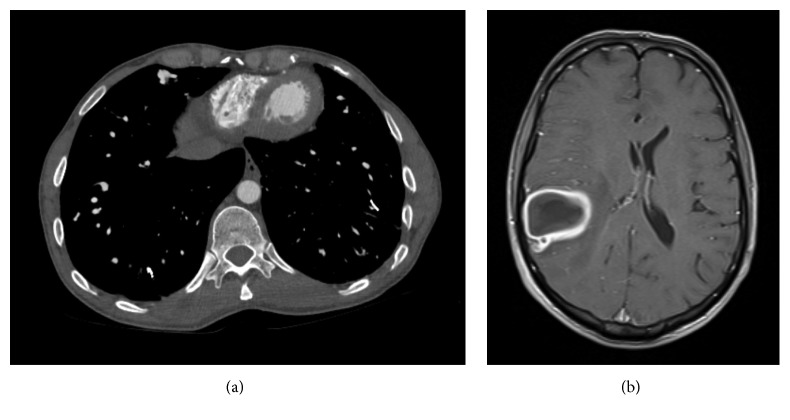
Computed tomography of chest showing multiple PAVMs in right and left lobes (a). T1 weighted postcontrast cerebral MRI showing 4.7 × 3.9 × 5.5 cm ring enhancing lesion located in the right parietal lobe with surrounding edema (b).
